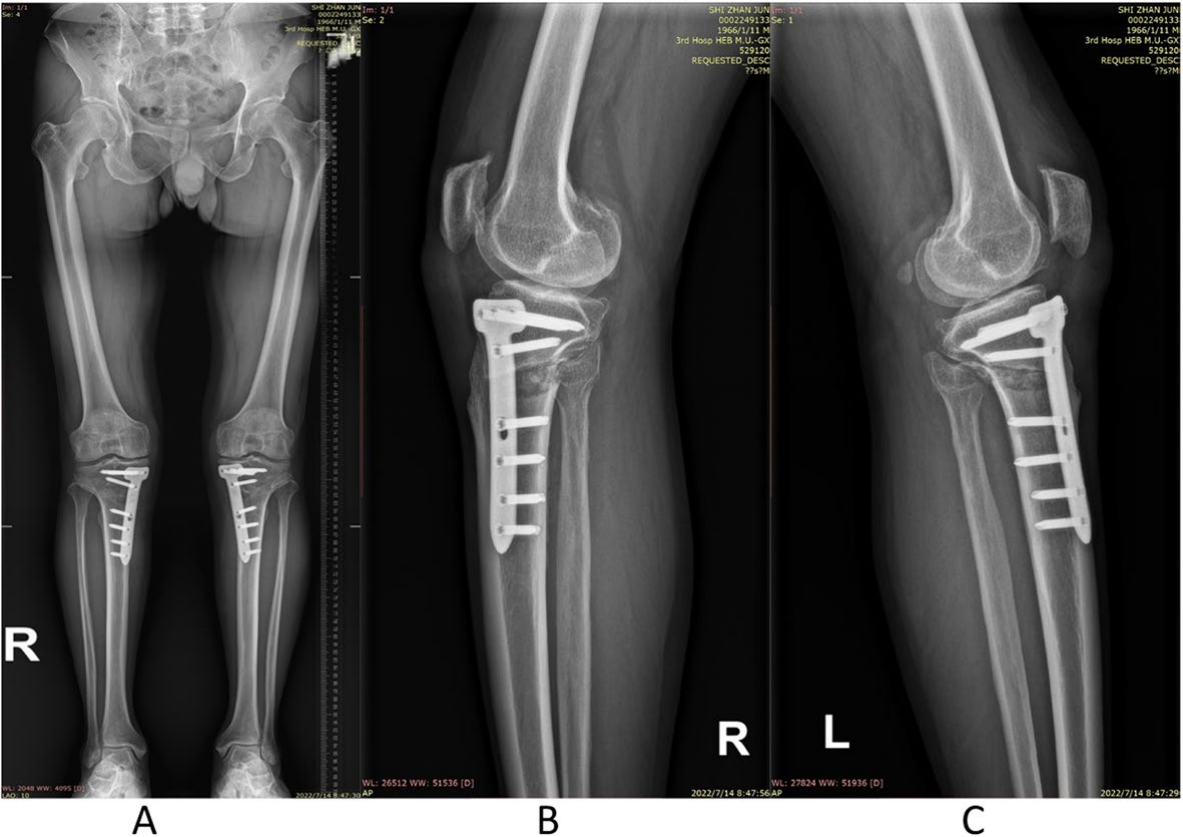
A-C Weight-bearing full-length and lateral radiographs of the lower limb and knee lateral x-ray after bilateral THO
